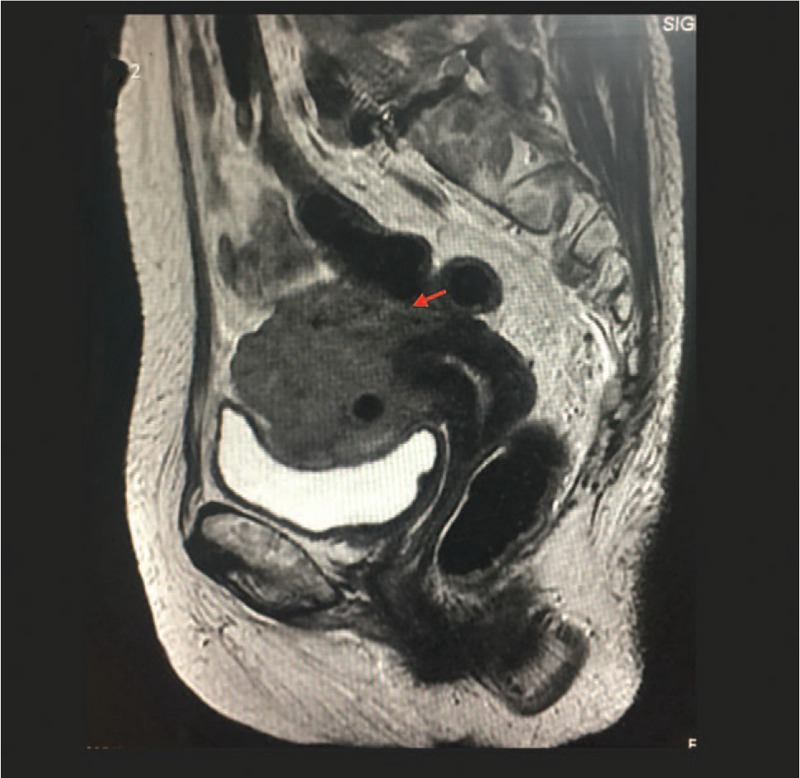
MRI reveals direct invasion of the rectal mucosa.
